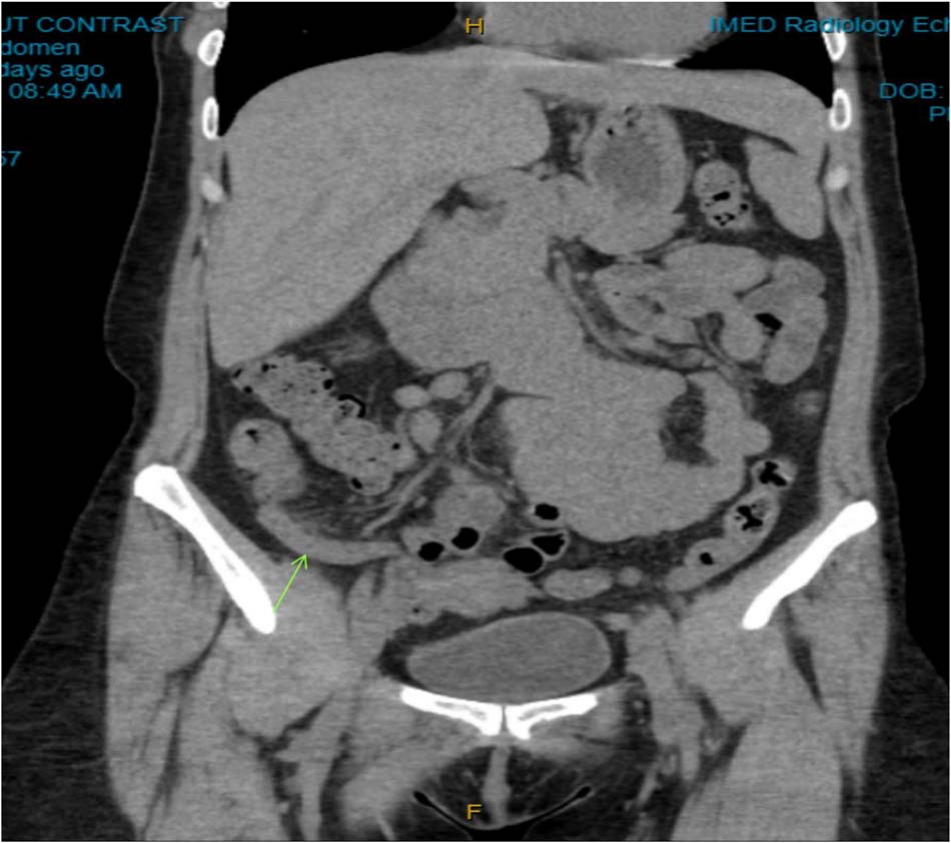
Coronal CT image. Dilated appendix with mild surrounding fat stranding.
